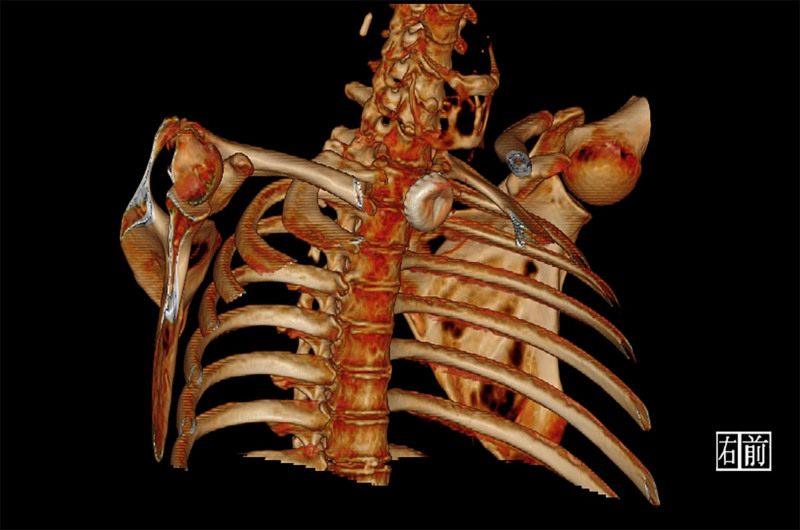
Chest CT 3-dimensional reconstruction demonstrated a metallic bottle cap impacted anterior to the T1–T2 vertebral bodies, with central concavity of the bottle cap (arrow). CT = computed tomography.
